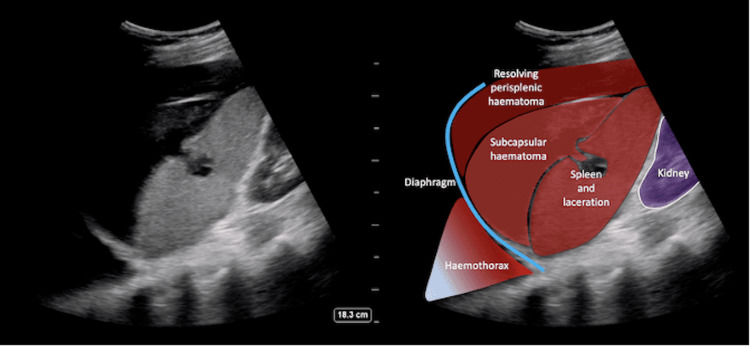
Splenic rupture FAST FAST left upper quadrant (LUQ) view showing that there is heterogeneous predominantly hyperechoic perisplenic hematoma as well as subcapsular hematoma. The echogenic area in the mid portion of the spleen disturbing the usual homogeneous echotexture suggests a laceration [10]. DISCLAIMER: The FAST images provided are not the FAST images from our patient, but rather a positive FAST in the LUQ. Written permission from the original publishers was obtained to reproduce these images. FAST: Focused Assessment with Sonography in Trauma
